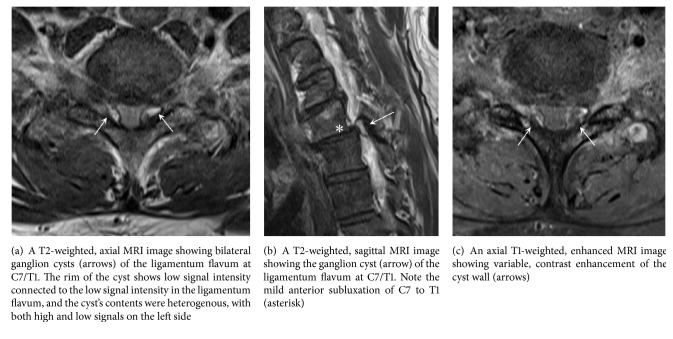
Magnetic resonance imaging (MRI) findings of bilateral ganglion cysts of the ligamentum flavum at C7/T1.
